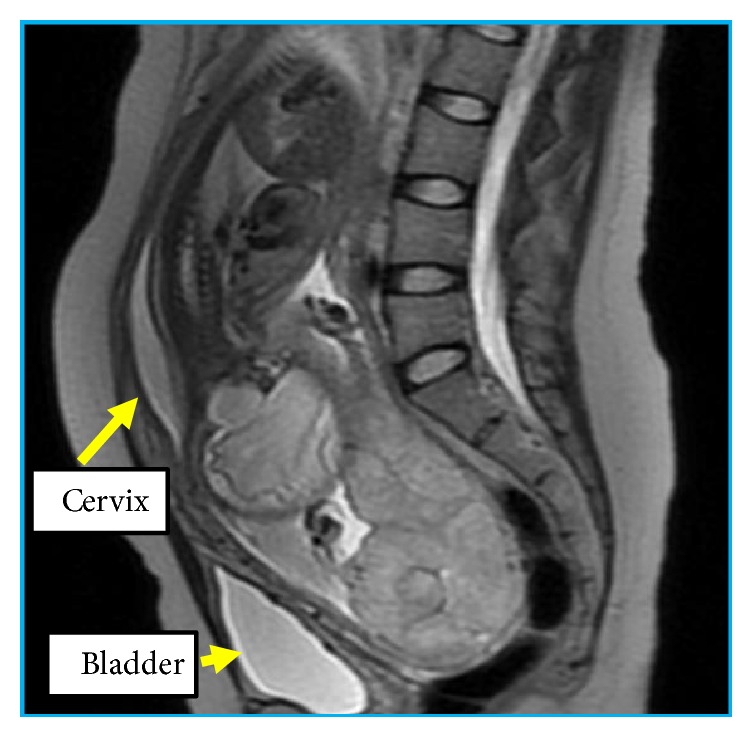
Magnetic resonance imaging scan at 31 weeks and 2 days of gestation.
